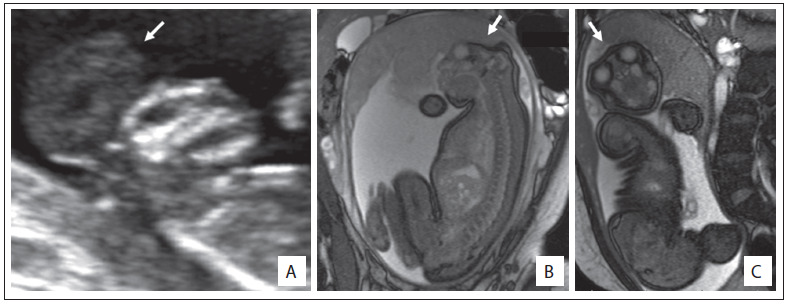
Two-dimensional ultrasound showing the profile of fetuses presenting exencephaly, i.e. the first stage of anencephaly (case 1). Note also the presence of a significant amount of brain tissue (A). Appearance of one of the fetuses (case 12) with anencephaly (see arrows) evaluated through magnetic resonance imaging (B and C).
